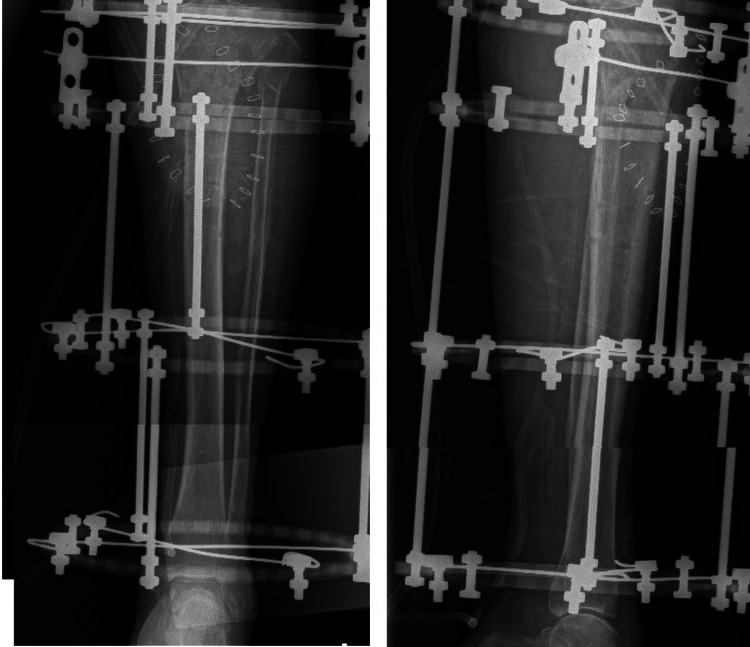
Plain radiographs showing anteroposterior and lateral views after external fixation.
